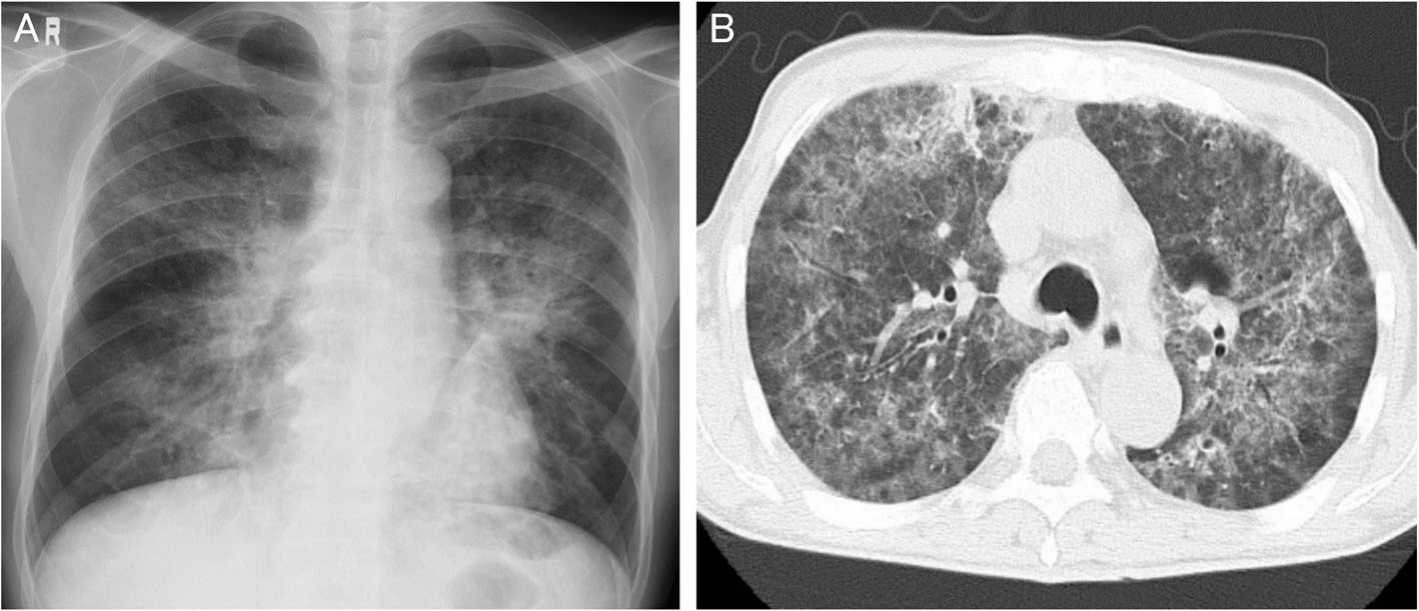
Chest radiographs. (A) Chest X-ray revealed infiltration shadows in bilateral middle and lower lung fields. (B) Chest computed tomography scan revealed infiltration and fine granular shadows
